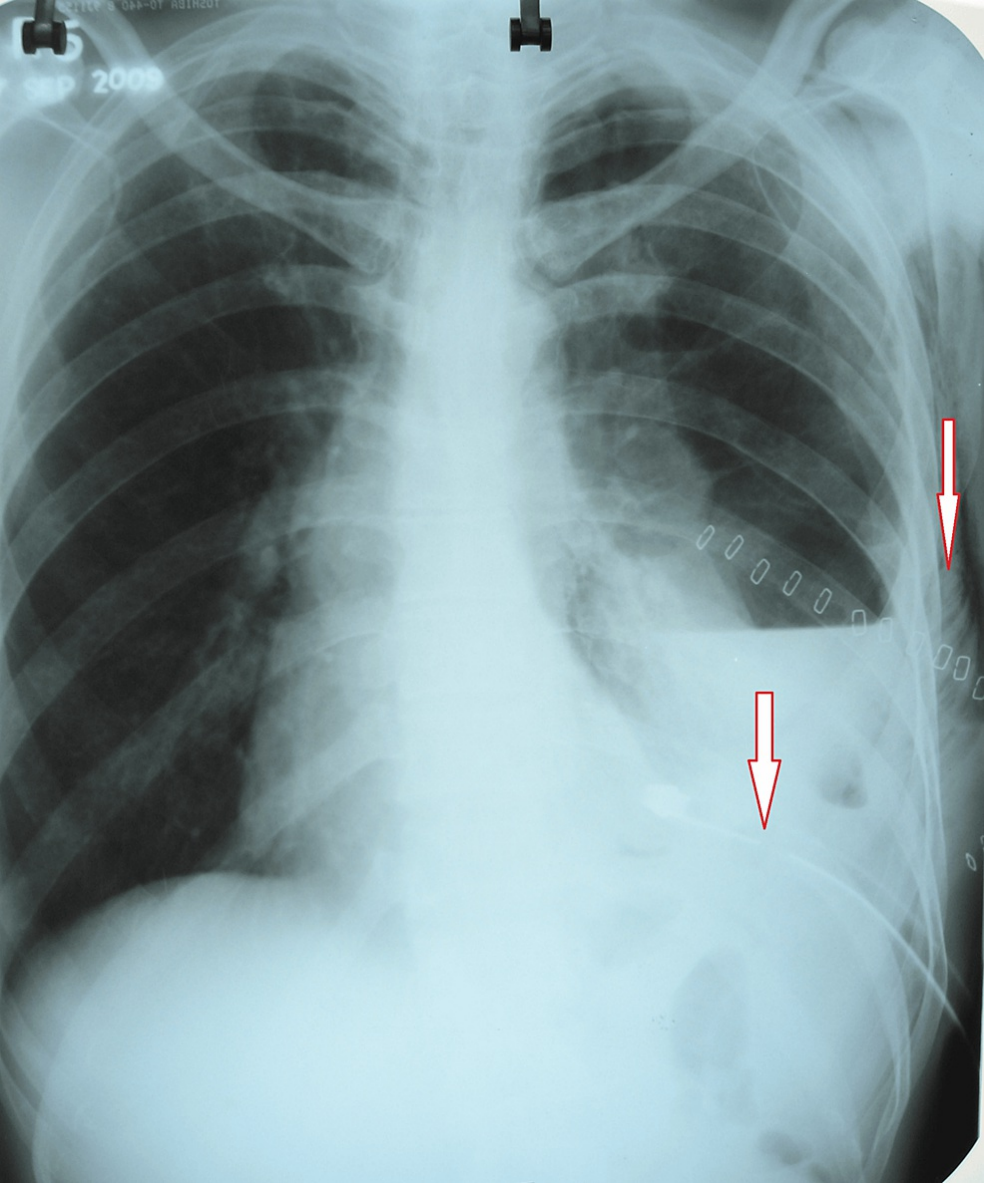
Postoperative chest X-ray showing intercostal drainage in situ for loculated pyopneumothorax with visible pleural thickening.
